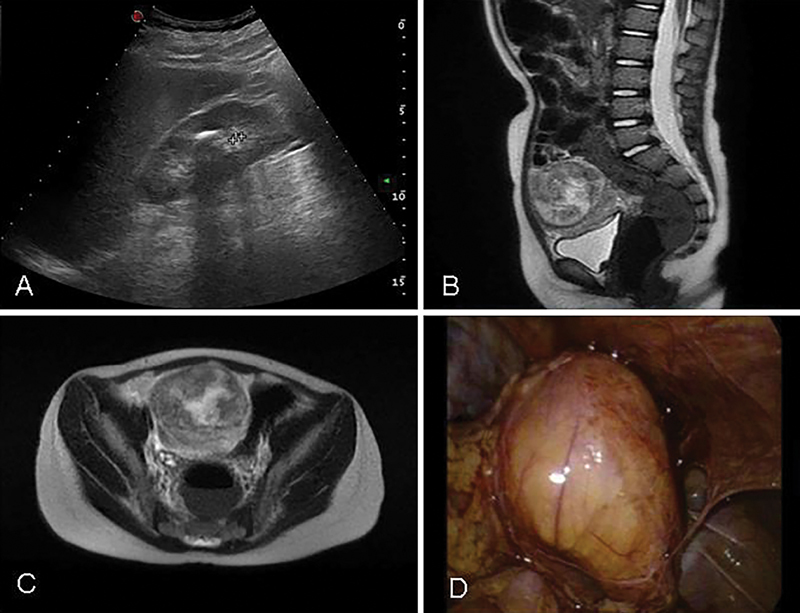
Abdominal ultrasound showing a 6 cm right-sided ovarian mass that was consistent with teratoma (
